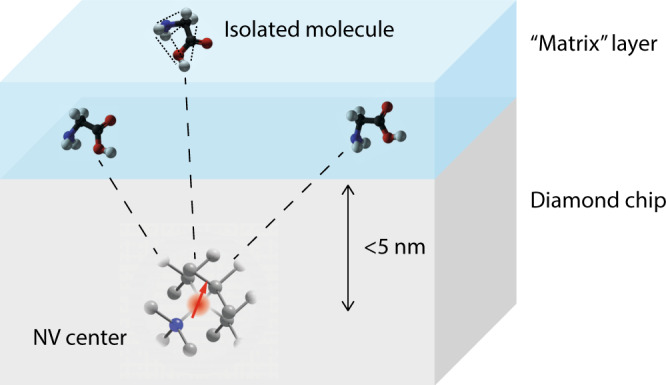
Proposed strategy for single-molecule MRI. A few molecules of interest are immobilized on a diamond surface containing <5-nm-deep NV centers. Molecules are protected by a spin-free “matrix” layer (blue shading). The global position and conformation of molecules are imaged by our weak-measurement localization scheme (dashed lines), while precise local distances between nuclei are measured using two-dimensional NMR (dotted lines). Computational analysis is used to extract three-dimensional atomic coordinates.
